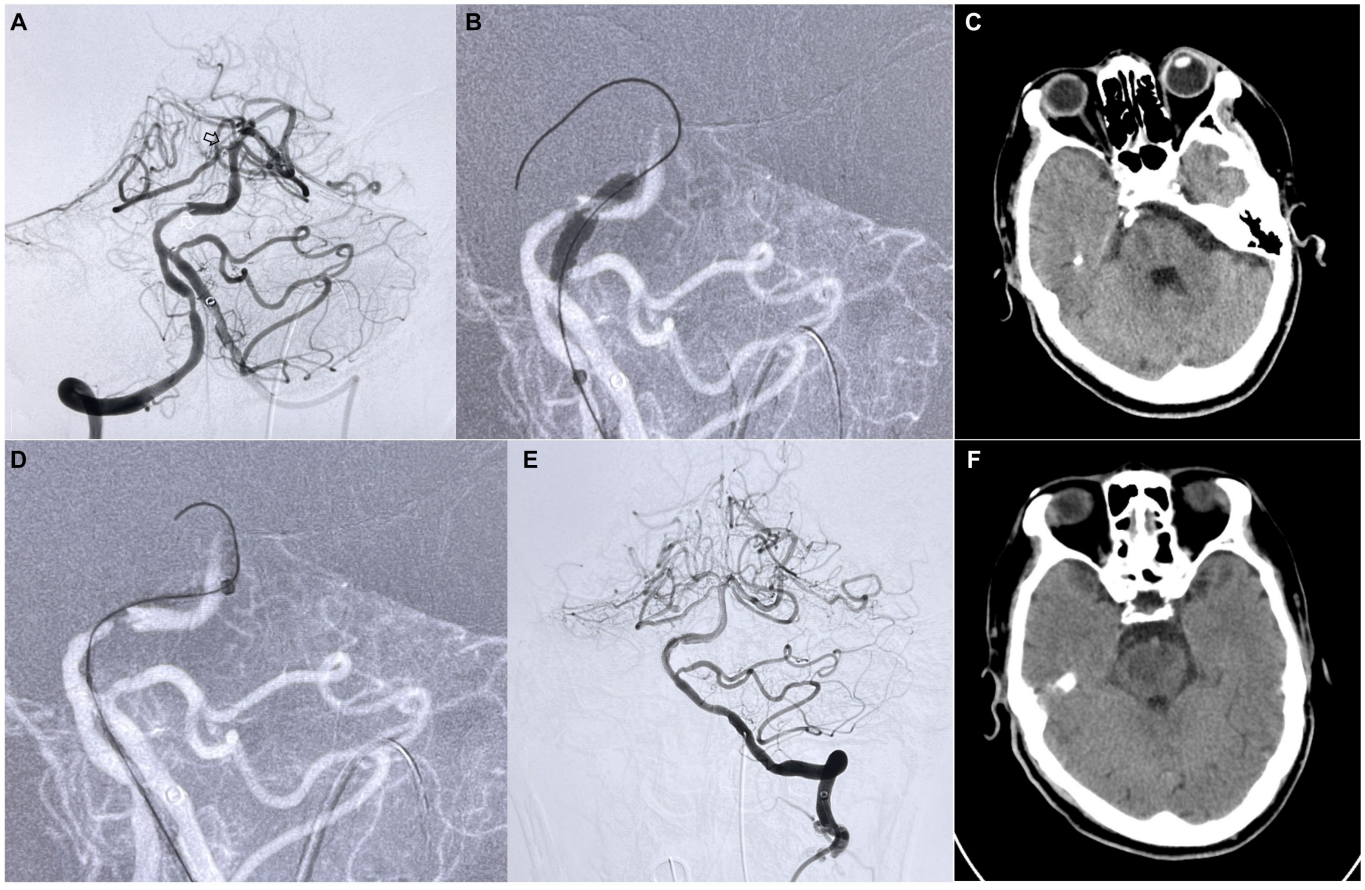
Patient suffered a sudden weakness of both lower limbs and loss of consciousness for a last-known normal time of 17 h and was admitted to the hospital. (A) Angiography shows an ICAS-related stenosis located at the proximal of the basilar artery (white arrow) accompanied by a distal thrombosis occluded at the top of the basilar artery (black arrow). (B,D) The standard BAT technique was applied to this patient for crossing the narrow site proximal to the intermediate catheter. (E) After thrombectomy for the distal acute occlusion using a Solitaire stent, the proximal stenosis was only treated with balloon dilation to achieve a TICI 2b recanalization. (C,F) Due to the long-term occlusion, compared with preoperative CT (C), postoperative CT (F) shows that the infarction area focus of the midbrain and pons was larger than before. No signs of intracranial hemorrhage or massive infarction were found after BAT and thrombectomy.
